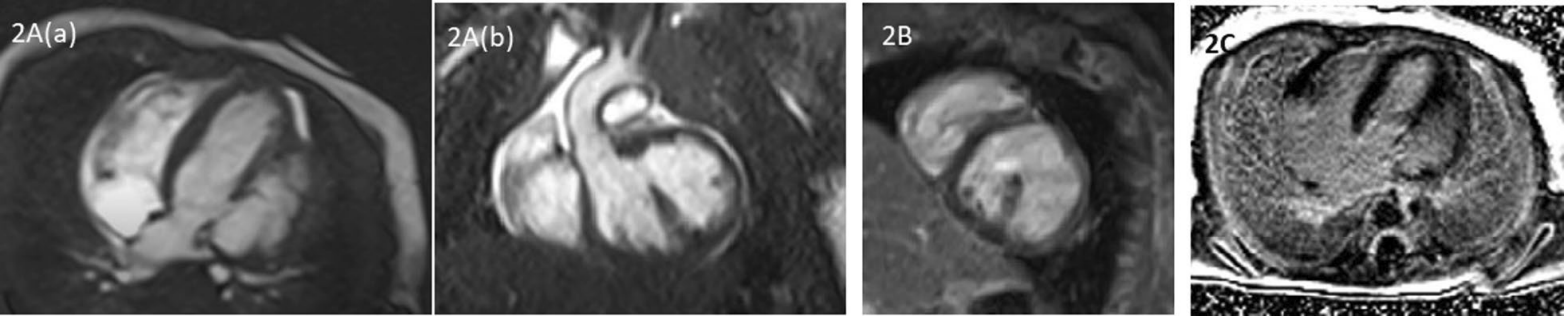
A: Apical 4 chamber (a) and LVOT (b) view showing double-chambered left ventricle. B: Early gadolinium image showing double-chambered LV with no evidence of thrombus. C: Late gadolinium image with no evidence of myocardial enhancement
